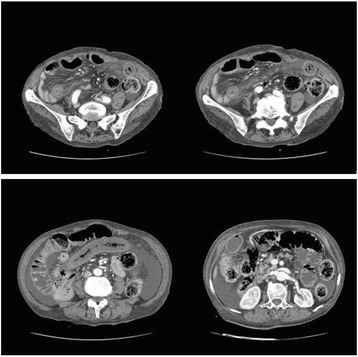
Angio-CT, a case of NOMI: Radiologic Science Department–AOUP Palermo
